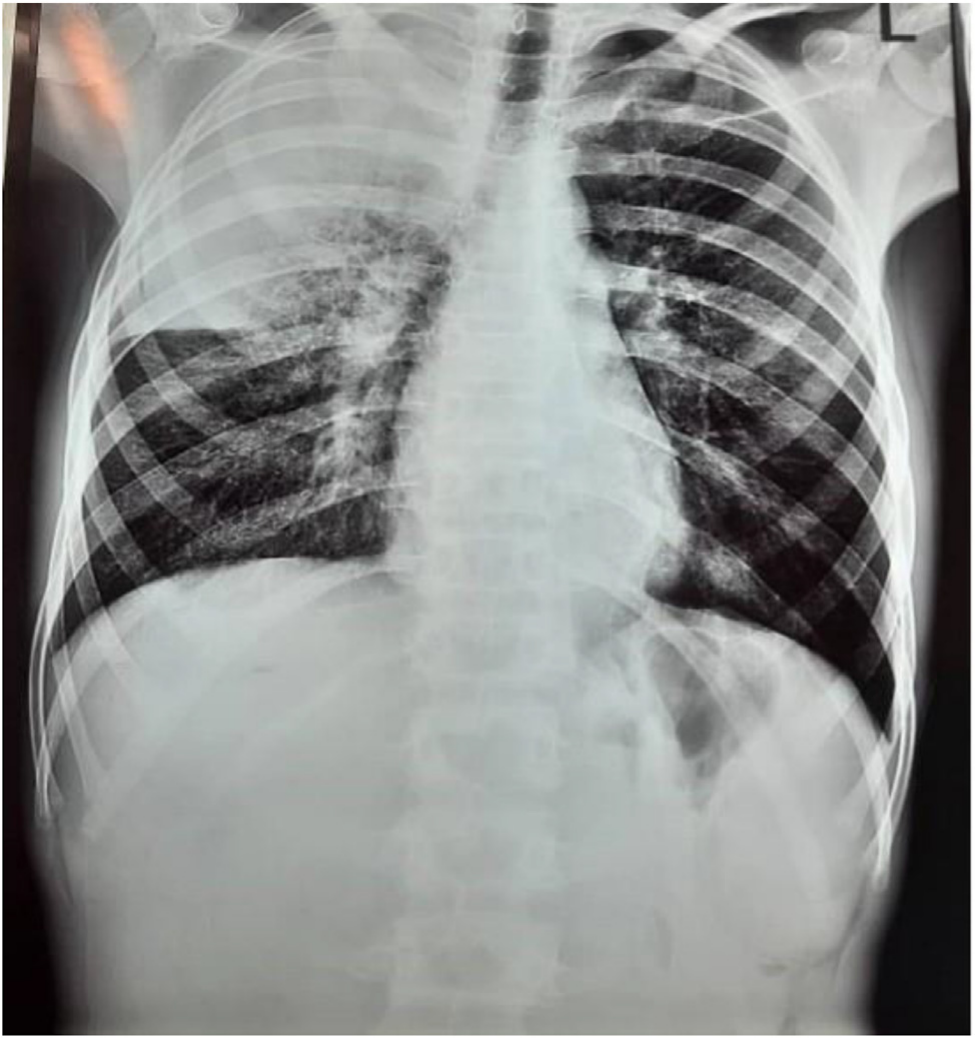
Chest X-Ray revealed Consolidation in right upper lob. Most probably due to local Pneumonia.
